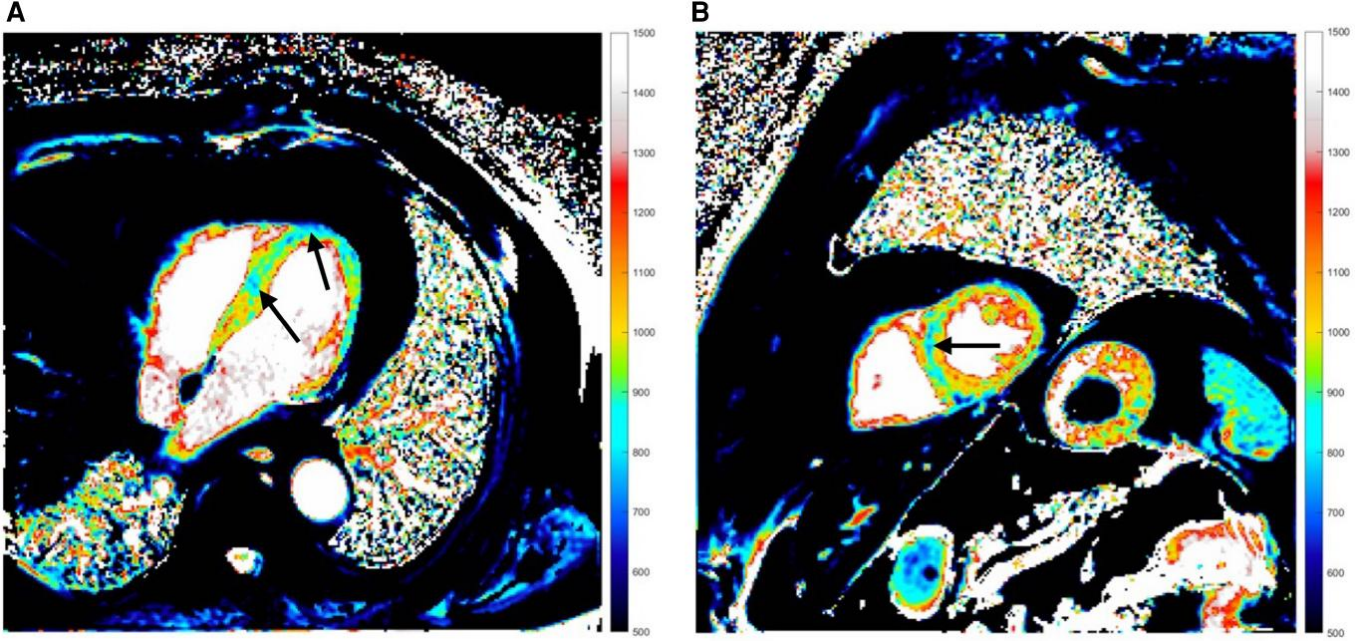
Follow-up cardiac MRI native T1 long and midventricular short axis views. Compared with normal T1 values, unusually low T1 signals were observed in certain areas, highlighted by black arrows, suggestive of iron deposition in the myocardium and liver (MRI, magnetic resonance imaging).
